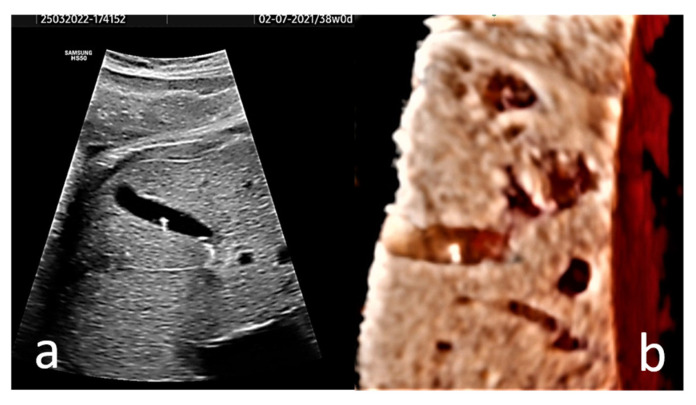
(a) Axial section of the gallbladder of a 38 gestational weeks fetus. The hyperechogenic spots are well-defined and do not present distal shadowing; instead they show comet tail artifact. (b) A 3D rendered image of the fetus’ gallbladder is provided. A Voluson S8 ultrasound machine with abdominal convex array volume probe RAB6-RS (GE Healthcare, Zipf, Austria) was used.
